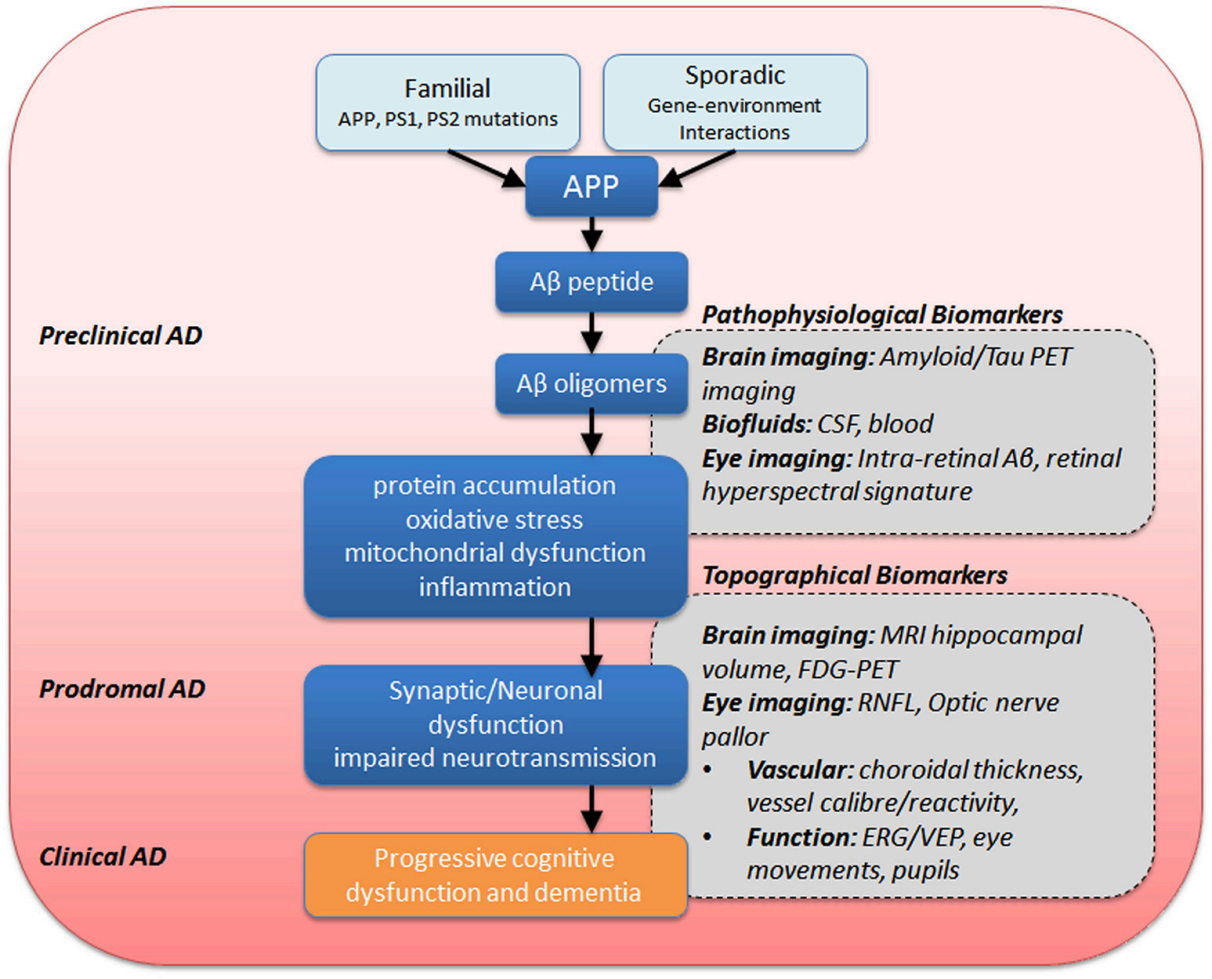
Summary of eye and brain biomarkers of AD. APP, amyloid precursor protein; PS1/PS2, presenilin-1 −2, ERG, electroretinography; CSF, cerebrospinal fluid; MRI, magnetic resonance imaging; PET, positron emission tomography; FDG-PET, fluorodeoxyglucose-PET; RNFL, retinal nerve fiber layer; VEP, visual evoked potential.
